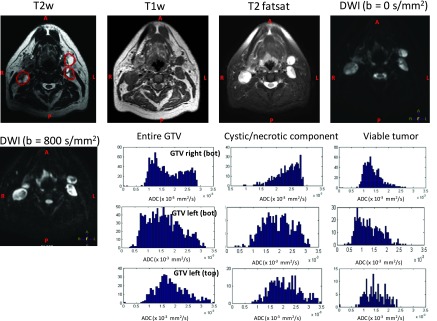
GTV nodal volume shown on T2w, T1w, T2 fatsat, DWI (*b* = 0 and 800)
